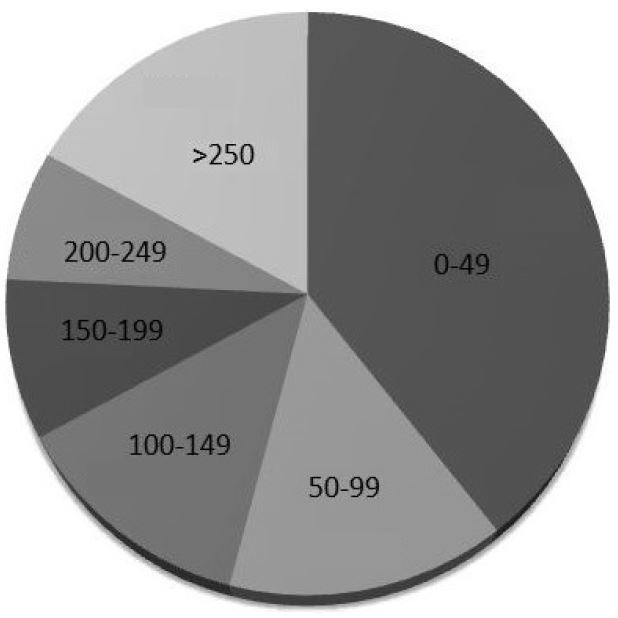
Prior ultrasound experience of the sonographers who performed the bedside thoracic ultrasound examinations.
